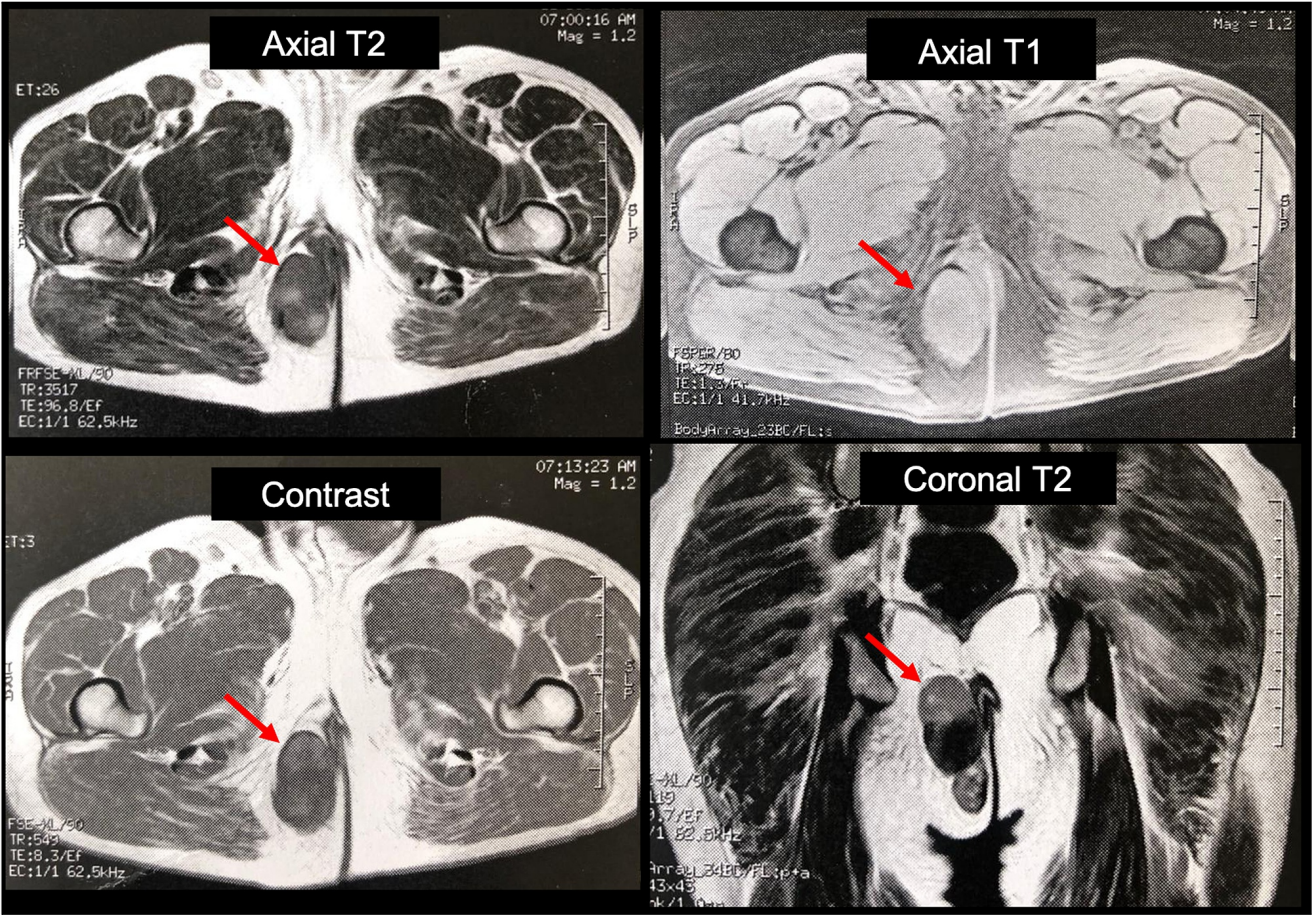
Contrasted MRI Mass with lobulated contours in the right ischioanal base whose dimensions were 36 x 65 x 74 mm, in close contact with the external anal sphincter without contact with the skin of the gluteal fold (red arrow) MRI: magnetic resonance imaging
